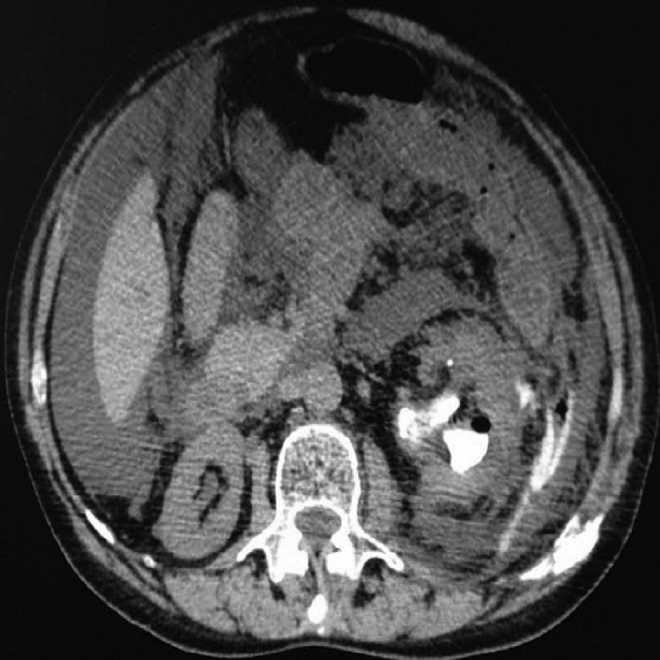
Postoperative CT scan showing intraperitoneal fluid collection.
